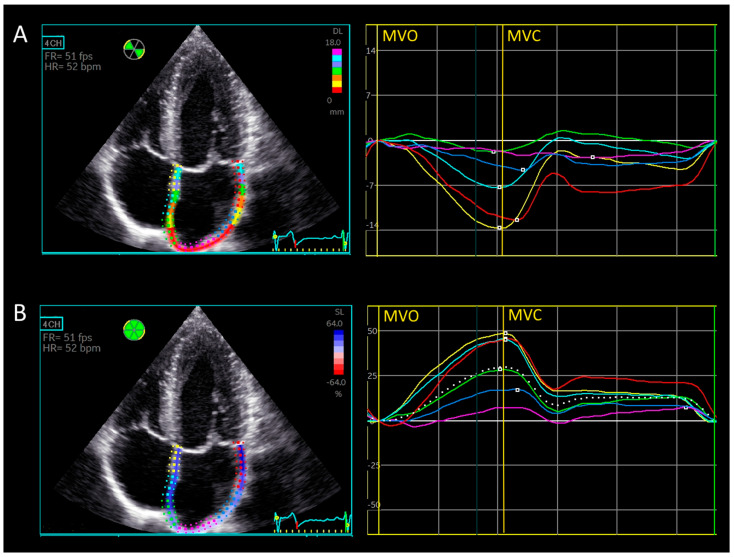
Longitudinal displacement and strain of the left atrium. (A) Apical 4-chamber view, longitudinal displacement curves from six segments are shown. MVO—mitral valve opening. MVC—mitral valve closure. (B) Apical 4-chamber view. Regional and global longitudinal strain from left atrium is shown. MVO—mitral valve opening. MVC—mitral valve closure.
